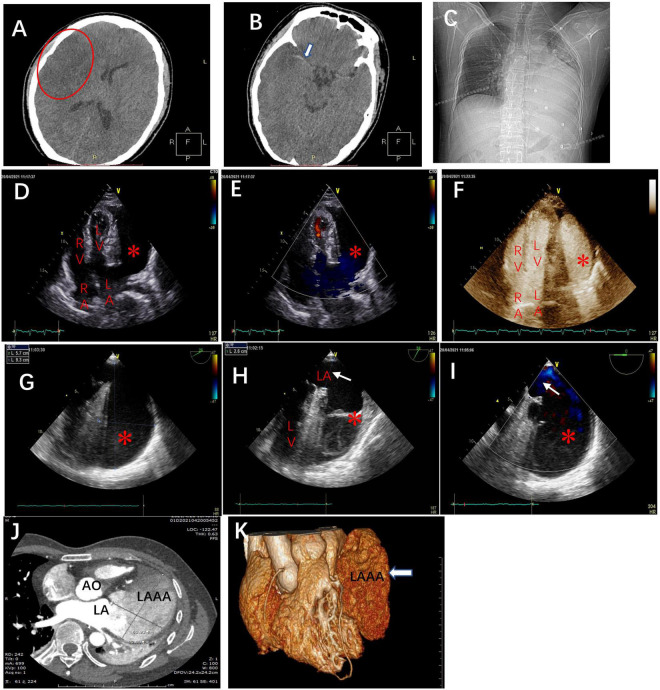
The brain computed tomography scans at admission revealed (A) massive cerebral infarction in the right-sided frontotemporal insula (red circle) and (B) the basal ganglia area with right middle cerebral artery thrombus (white arrow). (C) The chest X-ray in the cardiology department demonstrating the abnormal left heart border. Transthoracic echocardiography (TTE), apical four-chamber view (D), and the view with color doppler (E) revealing an echo-free cavity (red star) adjacent to and compressing the LV and the probable communication of the cavity with the LA; TTE with contrast showing the contrast filling in the LA and the cavity simultaneously without filling defect in the cavity (F); TEE, the mid-esophageal 2-chamber and LAA view demonstrating a giant cavity (red star) measuring 9.3 cm × 5.7 cm (G) and its connection to LA *via* a 2.6 cm-wide orifice (white arrow) (H) and the to-and-fro blood flows between LA and the cavity (red star) through the orifice (white arrow) (I). Axial computed tomography scan (J) and 3D reconstruction (K) demonstrate a 9.3 cm × 6.4 cm × 3.8 cm giant LAAA. AO: aorta; RA: right atrium; LA: left atrium; RV: right ventricle; LV: left ventricle.
